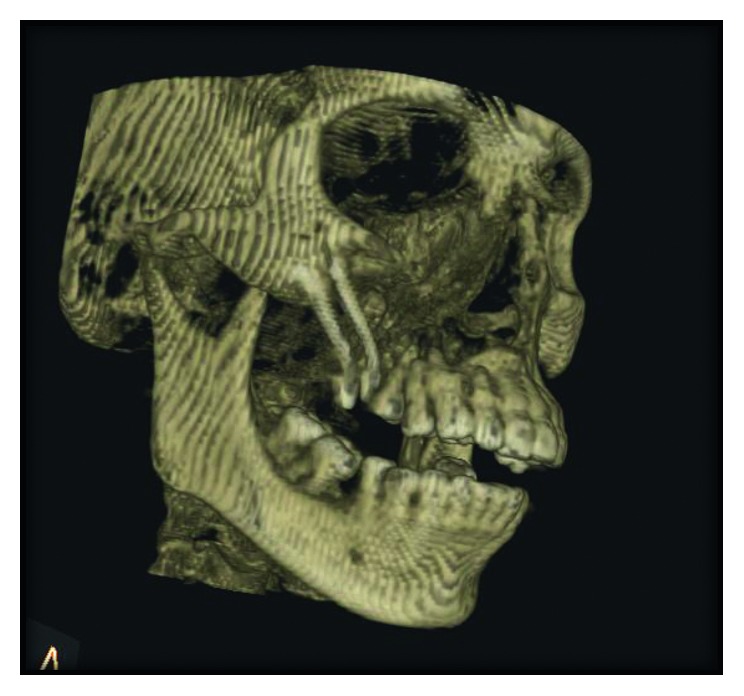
Postoperative CT shows apical two-thirds of zygomatic implant has engaged the medullary and cortical regions of the zygomatic bone.
